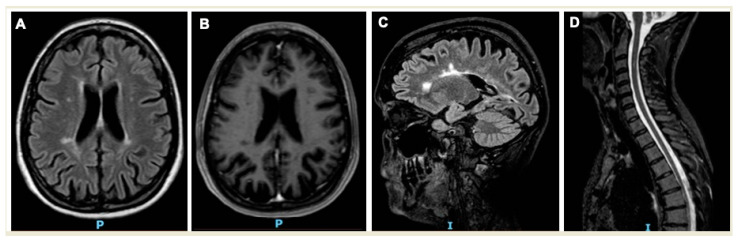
Brain and spine MRI at diagnosis of MS. Legend: the figure shows four MRI sequences (A–D). Explanation below. (A) Axial T2-weighted using a fluid attenuated inversion recovery (FLAIR), sequences of the brain MRI show typical periventricular hyperintense lesions due to the demyelination of the white matter. (B) The hyperintense lesions observed in sequence A appear hypointense in axial T1-weighted sequences of the brain MRI, due to axonal destruction and irreversible damage (also called “black holes”). (C) Sagittal T2-weighted using a fluid attenuated inversion recovery (FLAIR), sequences of the brain MRI show periventricular hyperintense lesions along the axis of the medullary veins, perpendicular to the body of the lateral ventricles and of the callosal junction (also called “Dawson’s fingers”). (D) Sagittal T2-weighted sequences of the spine MRI show a hyperintense demyelinating lesion at C3 level.
